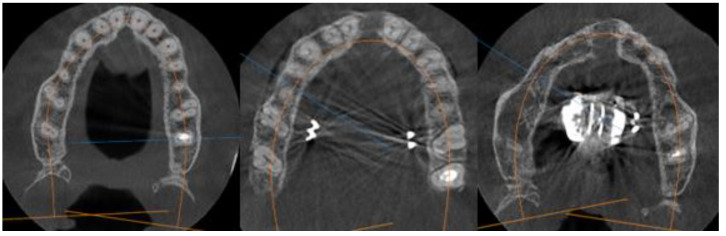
Cross sectional CBCT images before and after palatal split with MARPE device.
